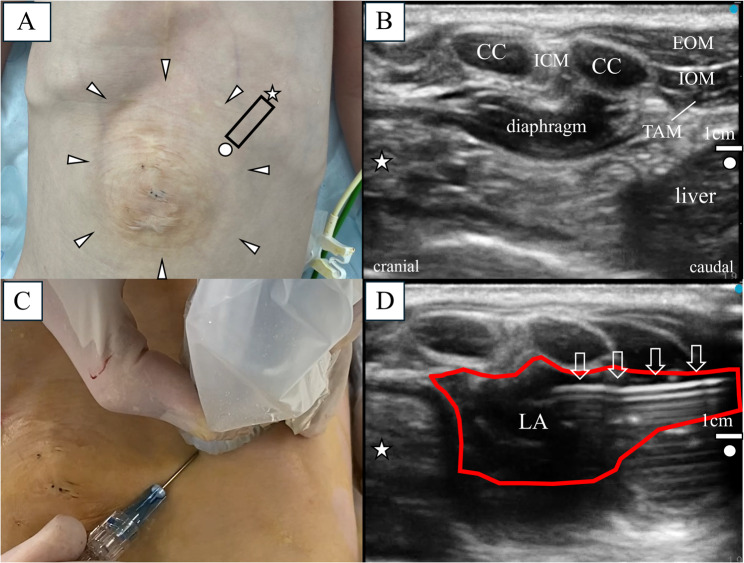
Ultrasound-guided upper abdominal wall block performed as an alternative to rectus sheath block. Because the rectus abdominis muscle structure was absent, an alternative upper abdominal wall block was performed instead of rectus sheath block. Rectangles indicate the position of the ultrasound probe. White stars and circles indicate the correspondence between the ultrasound probe and the orientation in the ultrasound images. A and B While checking the three-layer structure of the transverse abdominis, internal oblique, and external oblique muscles, the linear ultrasound probe was slid to the position where the internal oblique muscle was attached to the costal arch. The white triangles and rectangle indicate the area where the rectus abdominis muscle was absent after omphalocele repair and the position of the linear ultrasound probe, respectively. C and D 10 mL of 0.13% ropivacaine was injected into each side of the layer between the internal oblique and transverse abdominis muscles. This total dose was 1.68 mg/kg ropivacaine, which is below the maximum recommended dose for a pediatric patient in this case. The local anesthetic spread beyond the back of the ribs to the cranial side of the costal arch, pushing down the diaphragm [13, 14]. The arrow and red box indicate the needle and the spread of the administered local anesthetic, respectively. CC: costal cartilage, ICM: intercostal muscle, EOM: external oblique muscle, IOM: internal oblique muscle, TAM: transverse abdominis muscle, LA: local anesthetic. Video Caption. Video: Ultrasound-guided upper abdominal wall block performed as an alternative to rectus sheath block. Because the rectus abdominis muscle was not identifiable, an alternative upper abdominal wall block was performed instead of rectus sheath block. While checking the three-layer structure of the transverse abdominis, internal oblique, and external oblique muscles, the linear ultrasound probe was positioned where the internal oblique muscle was attached to the costal arch. The needle was inserted via a long-axis in-plane approach between the internal oblique and transversus abdominis muscles, and 10 mL of 0.13% ropivacaine was injected into each side. This total dose was 1.68 mg/kg ropivacaine, which is below the maximum recommended dose for a pediatric patient in this case. The local anesthetic spread beyond the back of the ribs to the cranial side of the costal arch, pushing down on the diaphragm
